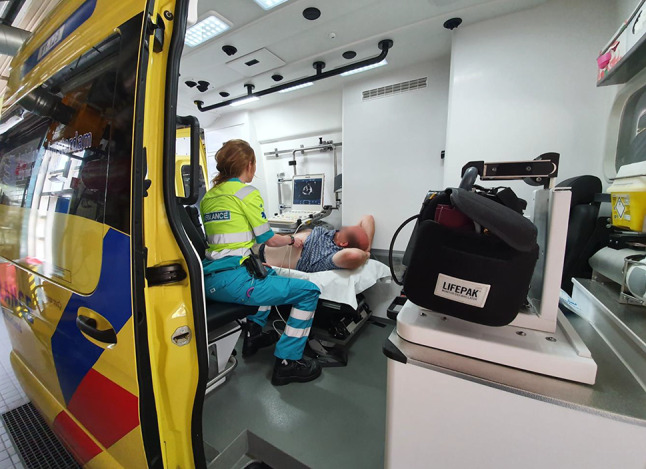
Portable ultrasound system mounted inside the ambulance enables diagnostic and therapeutic echocardiography (sonothrombolysis) during patient transfer to the PCI centre
